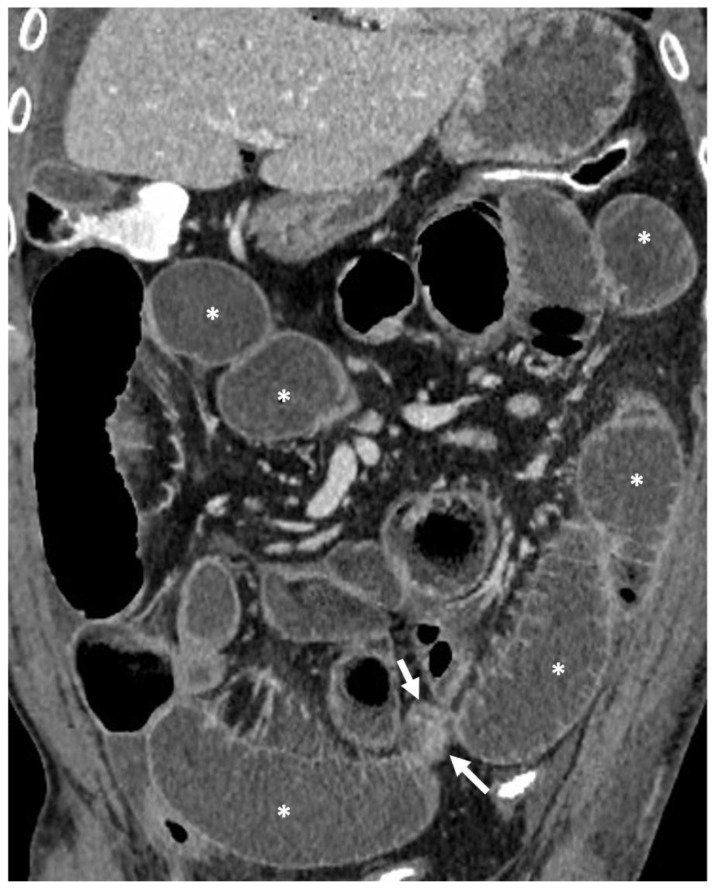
CT appearance of bowel obstruction caused by adhesive disease. Coronal CT image after contrast medium injection, performed at the emergency department, shows bowel dilatation (asterisks) upstream from the transition point (arrows). At the transition point, bowel loops appear angulated and distorted, suggesting the presence of adhesions (surgical confirmation).
